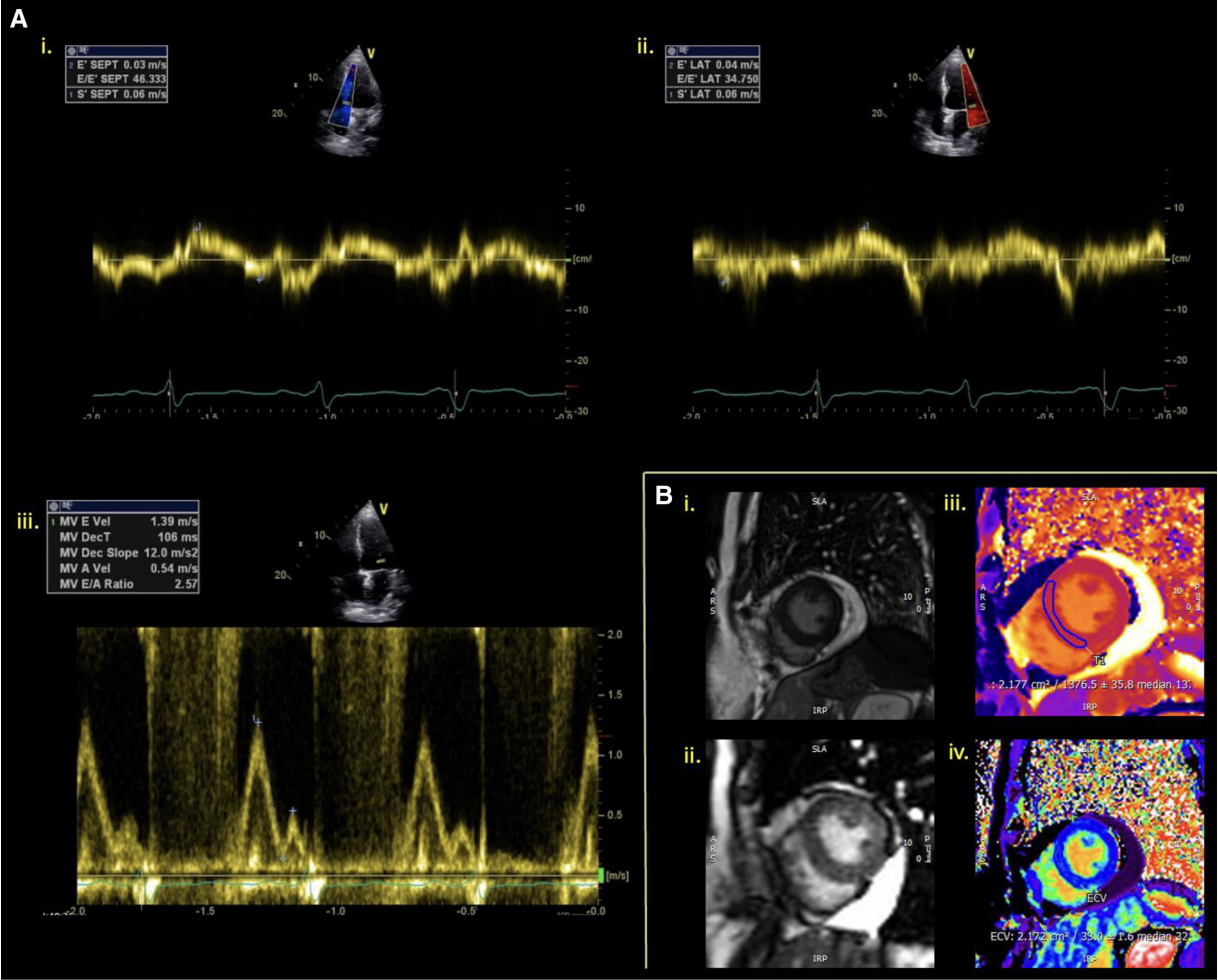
Echocardiogram demonstrating restrictive physiology in a 53-year-old female patient who had undergone radiotherapy 16 years previously for lung cancer. A, Transthoracic echocardiogram tissue Doppler of medial (i) and lateral (ii) mitral annuluses showing decreased e′ velocities. Pulsed wave Doppler of the mitral valve (MV) inflow showing E-wave>A-wave velocity (E Vel, A Vel) with a ratio of 2.57 with short deceleration time (DECT) of 106 ms (iii). Average E-wave/e′ of 40.54 and septal (SEPT) e′<lateral (LAT) E′ in keeping with restriction. B, Cardiovascular magnetic resonance imaging (CMR) (i) scout (ii) late gadolinium enhancement (LGE) imaging (iii) T1 MOLLI mapping and (iv) extracellular (ECV) mapping on 1.5T Scanner. Raised T1 at 1376 ms (normal values 970–1050 ms) and ECV at 32%.
